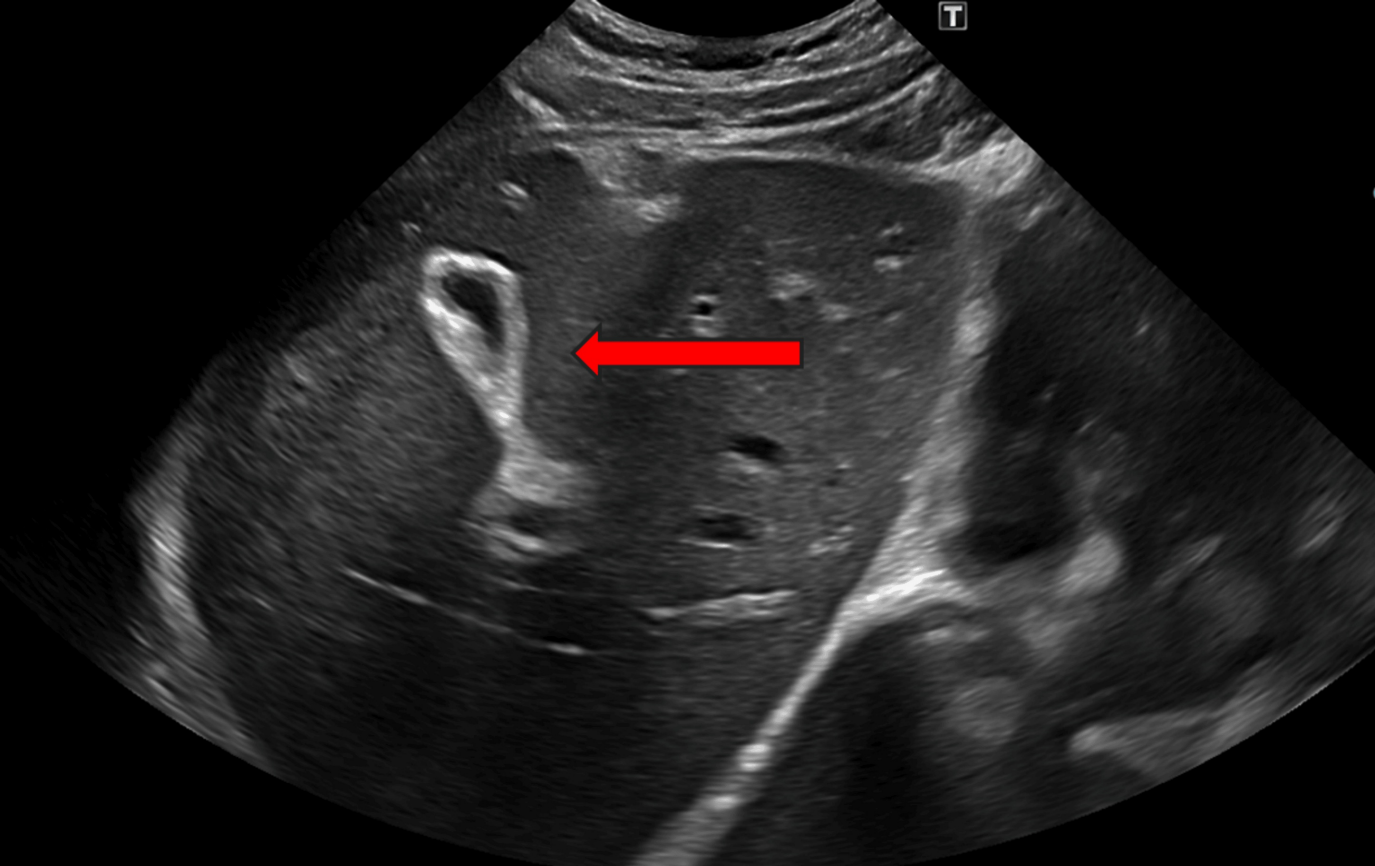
Ultrasound of the liver from the long axis showing hepatomegaly The red arrow points towards contracted gallbladder.
